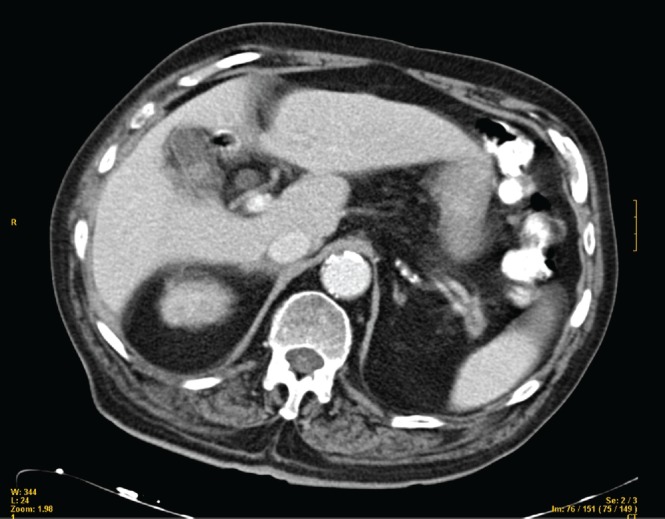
CT-scan at the end of chemotherapy treatment showing complete response.
